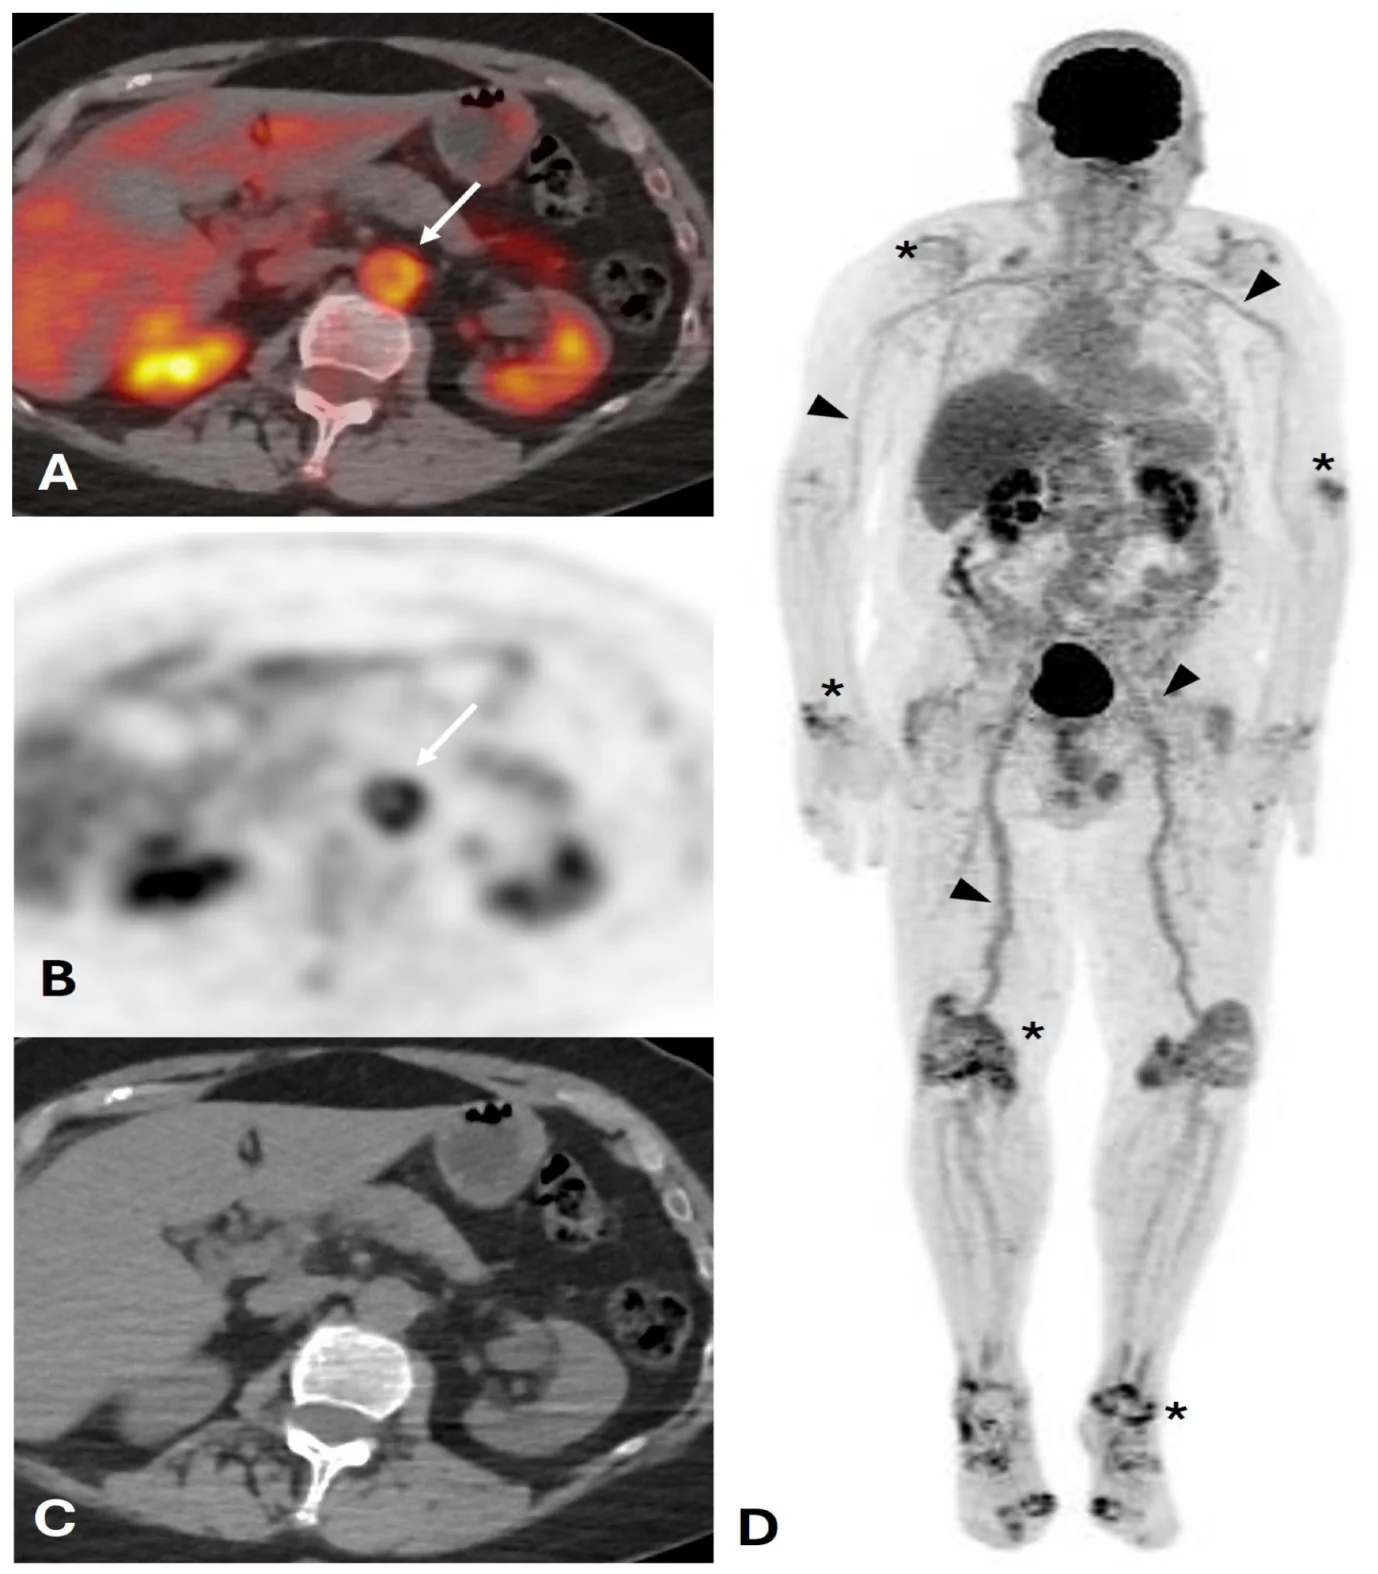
Whole body F-18 FDG PET/CT in a patient with known polymyalgia rheumatica evaluated for persistently elevated inflammatory markers. Axial fused (A), axial AC PET (B), axial CT without contrast (C) and whole-body AC PET MIP (D) images showing abnormal tracer uptake throughout the abdominal aorta (arrows) and to a lesser extent bilateral subclavian, axillary, brachial, iliac and femoral arteries (arrowheads) consistent with active vasculitis. In addition, heterogeneous moderate to intense abnormal tracer accumulation involving bilateral shoulders, elbows, wrists, hands, knees, ankles and feet (*) most consistent with active inflammatory polyarthritis.
